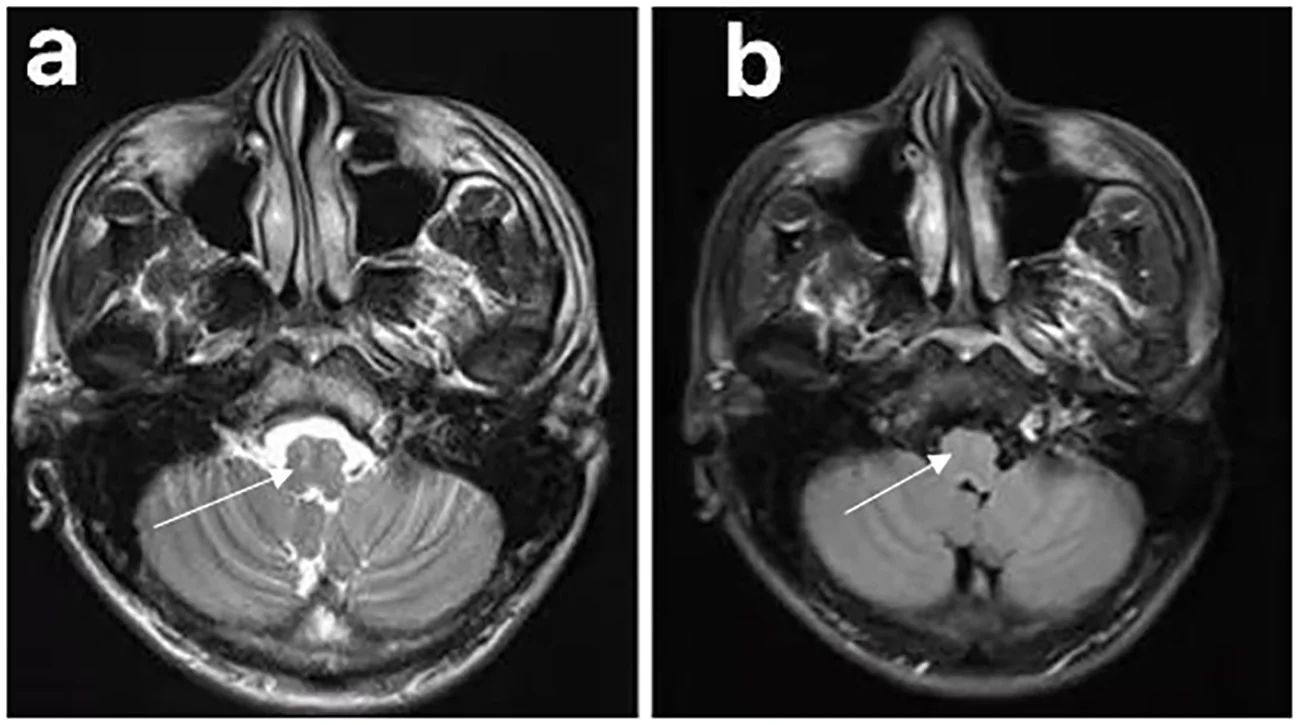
Brain magnetic resonance imaging (MRI) findings of patient 2. (A) T2-weighted imaging shows hyperintensity in the medulla oblongata. (B) T2-FLAIR imaging shows mild hyperintensity in the same region, with indistinct margins. No obvious abnormal enhancement is observed on contrast-enhanced scanning.
